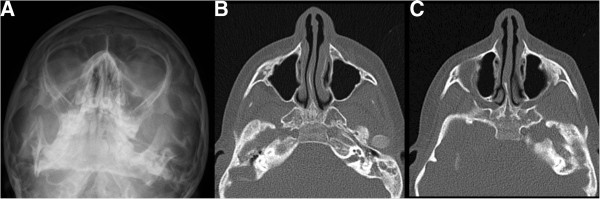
Zygomatic arch clefting of patient 6. Occipitomental view of the skull shows only rudimentary development of the zygomatic arch (A). Corresponding computed tomography shows large cleft in the right (B) and the left (C) zygomatic arch.
